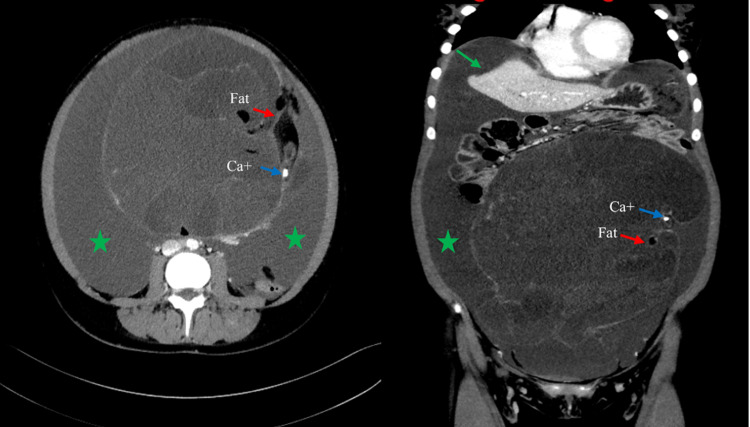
Axial and coronal CECT of the abdomen and pelvis showing a large multilocular cystic left adnexal mass with multiple fat containing lesion (red arrow) and foci of calcification (blue arrow) on the left aspect of the mass. Associated with massive ascites (green star) that is causing scalloping on the liver surface (green arrow). CECT, contrast-enhanced computed tomography
